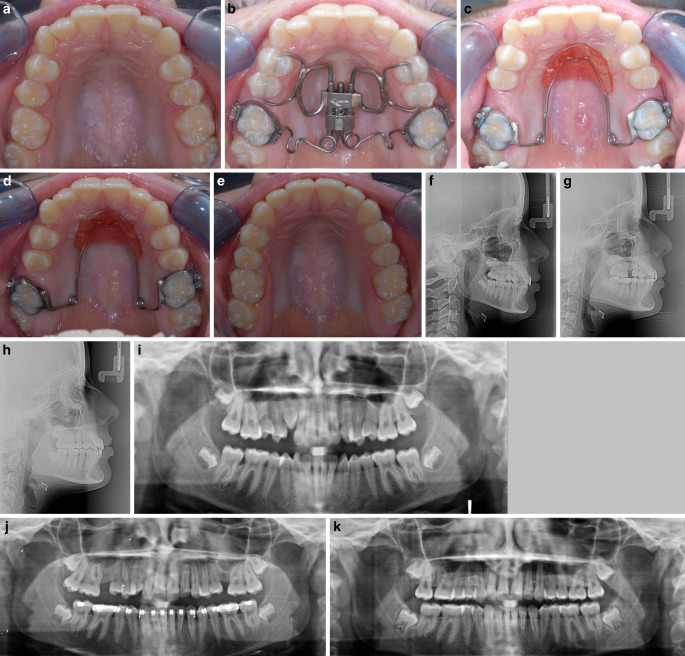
Skeletonized Pendulum K appliance, dental anchorage only. Patient example: female patient 14 years, 9 months of age, duration of molar distalization treatment 24 weeks. a Pretreatment: bilateral mesial migration of cuspids, premolars and molars. b Occlusal view immediately after skeletonized Pendulum K placement. c Occlusal view after completion of molar distalization: clinical assessment reveals bodily molar distalization. Retention using a combination of reduced Nance button and bi-helix. d Distal drift of cuspids and premolars after molar distalization und tendency of “self-alignment” of the dental arch. e Posttreatment: well-aligned dental arch. f–h Lateral cephalograms and i–k orthopantomograms at pretreatment, during treatment and posttreatment demonstrate bodily molar distalization Skelettierte Pendulum-K-Apparatur, nur dentale Verankerung. Patientenbeispiel: Patientin, 14/9 Jahre alt, Dauer der Molarendistalisationsbehandlung 24 Wochen. a Vor der Behandlung: bilaterale Mesialwanderung der Eckzähne, Prämolaren und Molaren. b Okklusalansicht unmittelbar nach Einsetzen der skelettierten Pendulum-K-Apparatur. c Okklusalansicht nach Abschluss der Molarendistalisation: Bei der klinischen Beurteilung zeigt sich eine körperliche Molarendistalisation. Retention mit einer Kombination aus reduziertem Nance-Pelotte und Bi-Helix. d Distaldrift der Eckzähne und Prämolaren nach Molarendistalisation und Tendenz zur „ Selbstausrichtung“ des Zahnbogens. e Nach der Behandlung: gut ausgeformter Zahnbogen. f–h Fernröntgenseitenbilder und i–k Orthopantomogramme vor der Behandlung, während und nach der Behandlung zeigen eine körperliche Molarendistalisation
